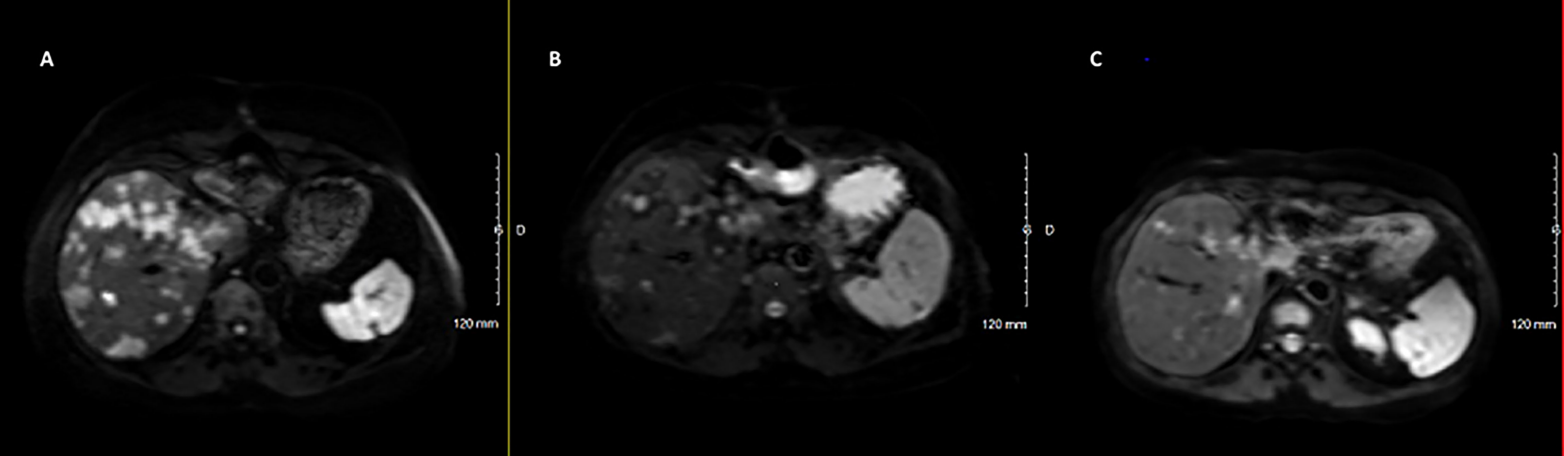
Radiological (MRI Diffusion) to CAPTEM and pasireotide. (A) June 2020, before CAPTEM; (B) December 2020, after 6 cycles of CAPTEM; (C) February 2022, pasireotide alone.
